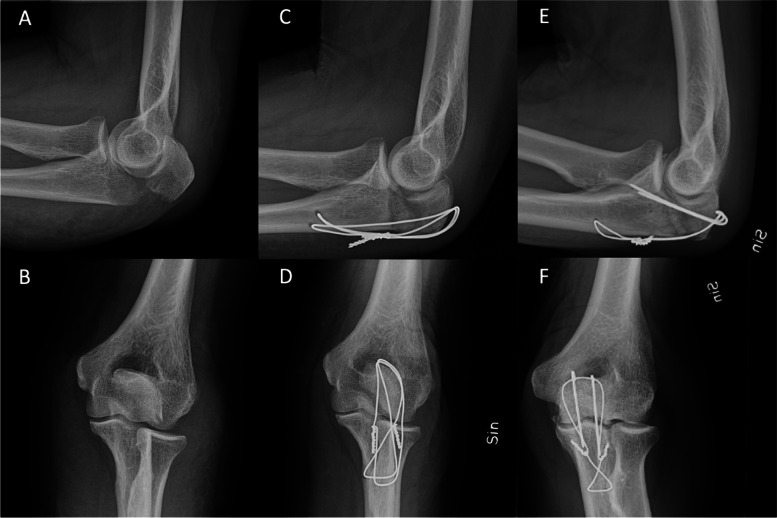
Fluoroscopy views of olecranon fractures. A, B Radiographs of olecranon type 2a fractures with a lateral (A) and an anterior–posterior view (B). Figure 1C and 1D shows radiographs following CF with a lateral (C) and an anterior–posterior view (D). E, F Radiographs following TBW with a lateral (E) and an anteriorposterior view (F)
